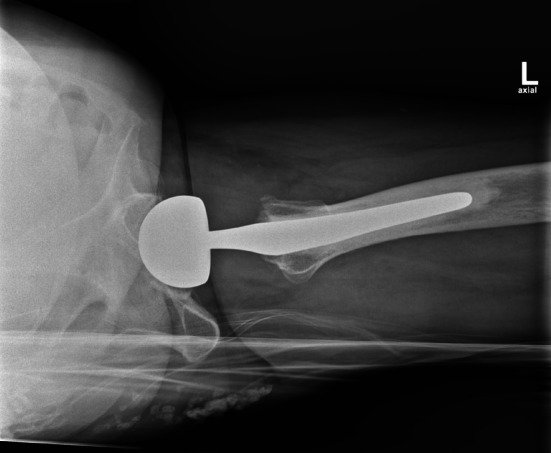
Axial radiographic view of the left hip demonstrating lobulated calcified subcutaneous deposits within the gluteal soft tissues, consistent with probable localized dystrophic calcinosis cutis at chronic interferon‐β‐1b injection sites.
